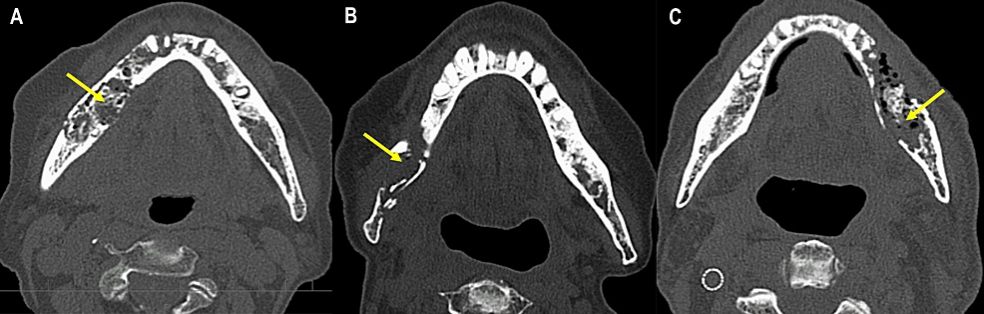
Axial computed tomographic images of Patient 1 (A), Patient 2 (B), and Patient 3 (C)
